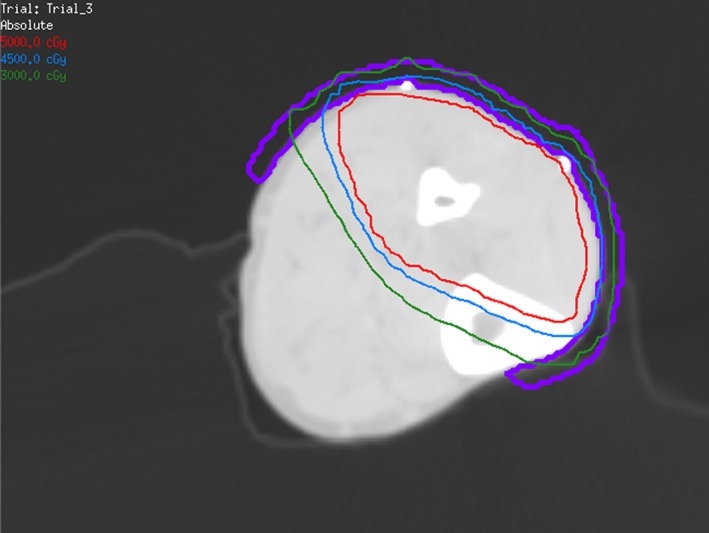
Axial computed tomography image showing radiotherapy isodose curves and bolus in the left lower leg.
